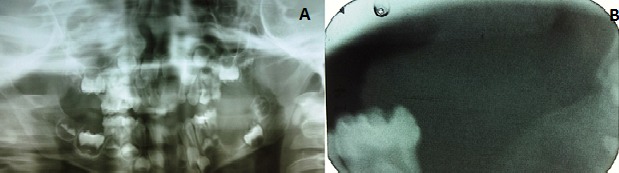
(A) OPG revealing an area of moderately well-defined radiolucency on the left posterior mandibular alveolar ridge distal to 75 with a discontinuous sclerotic margin; a speck of calcified mass was evident on the pericoronal region of the developing 36; (B) IOPA radiograph in the area revealed a soft tissue shadow and evidence of calcification with radiodensity similar to dentin
